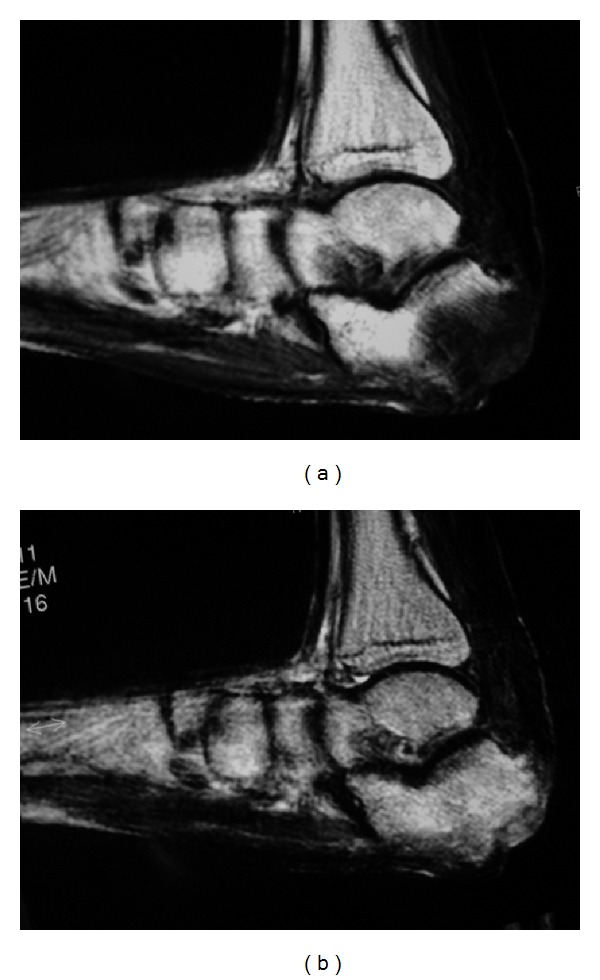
Magnetic resonance imaging of the preoperation. (a) T1-weighted sagittal imaging shows diffuse low signal intensity in posterior calcaneus. (b) T2-weighted sagittal imaging shows diffuse high signal intensity in the same region.
